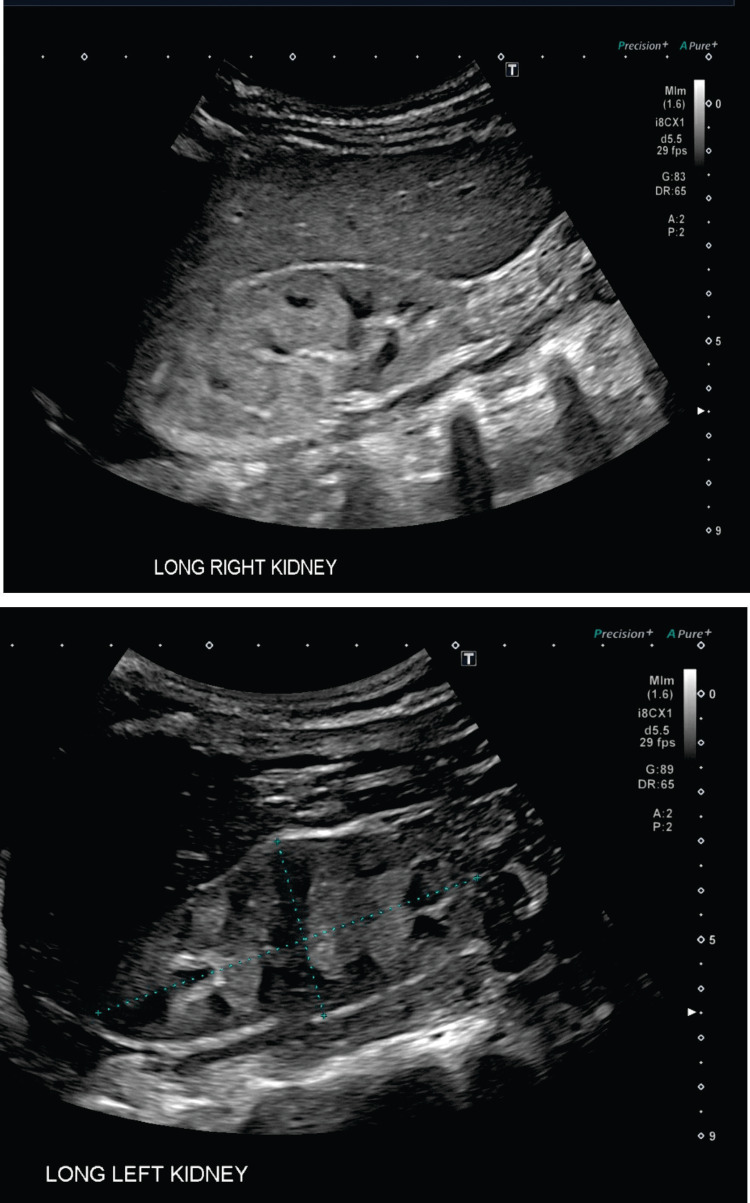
Renal ultrasound of native kidneys. The right kidney (upper) measures 7.7 × 3.0 × 4.3 cm. The left kidney (lower) measures 8.2 × 3.7 × 3.3 cm. The kidneys demonstrate increased parenchymal echogenicity with normal cortical thickness. There is no evidence of renal masses, calculi, or hydronephrosis.
